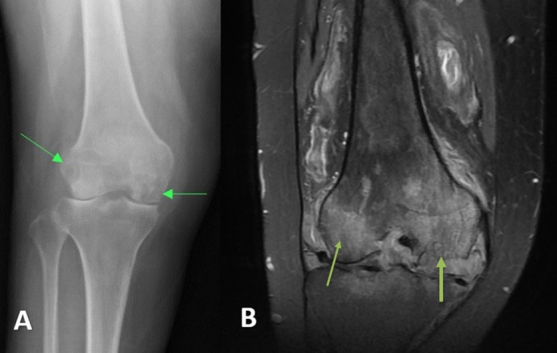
Case 2 (a) anterior–posterior right knee radiograph demonstrating subchondral lucency (green arrows), suggestive of advanced osteonecrosis and joint space narrowing consistent with arthritis. (b) T2-weighted sequence coronal section right knee MRI demonstrating cortical erosion suggestive of osteomyelitis (yellow arrows), with a mass-like synovial proliferation suggestive of atypical septic arthritis and full-thickness chondrolysis of the medial and lateral compartments.
